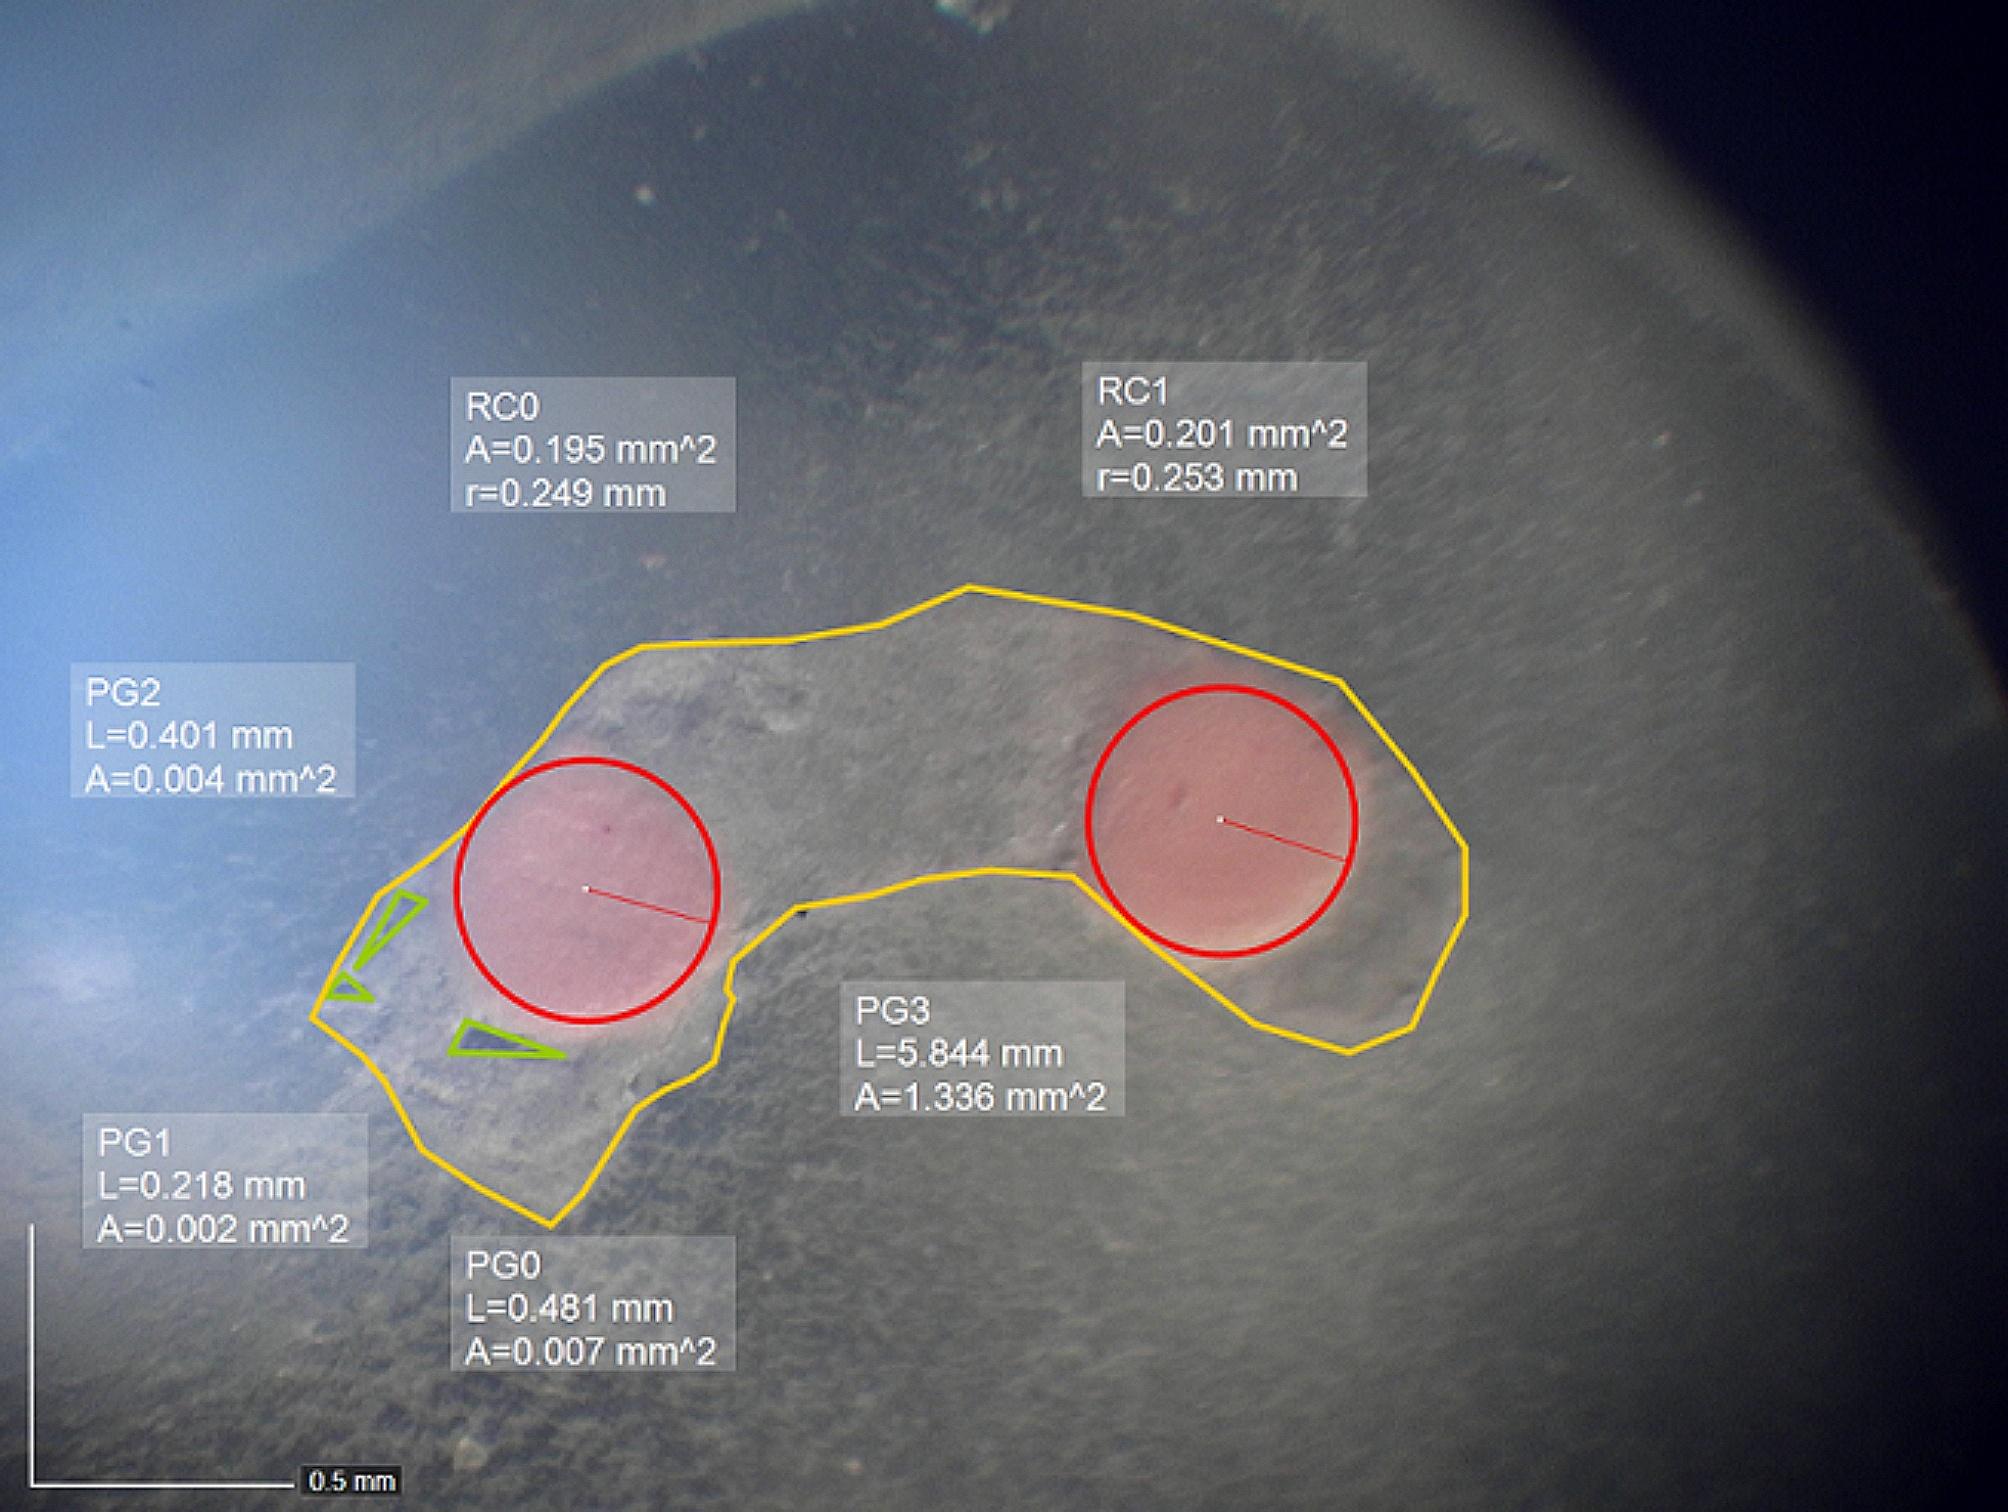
Measurements of the gutta-percha (red frame), sealer (yellow frame), and void (green frame) percentages in the middle third of a replica obturated with EndoSeal MTA without ultrasonic activation in 100x magnification
